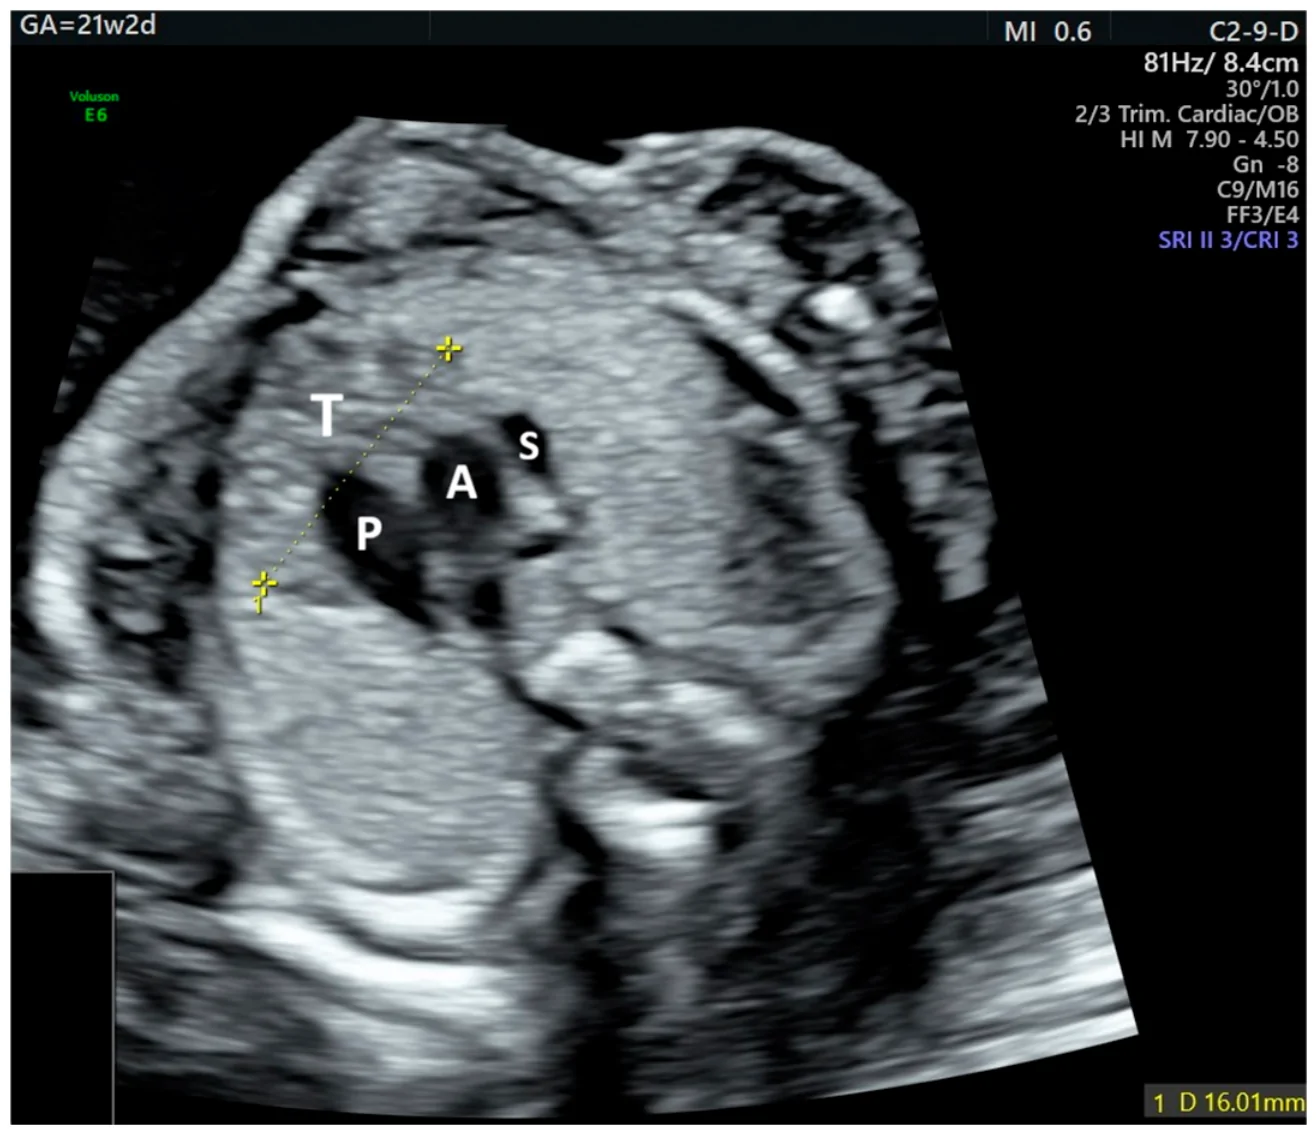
Representative ultrasound image demonstrating measurement of the maximum transverse diameter of the fetal thymus in the three-vessel trachea view. Calipers are positioned at the outer margins of the thymic tissue. T, thymus; A, aorta; P, pulmonary artery; S, superior vena cava.
